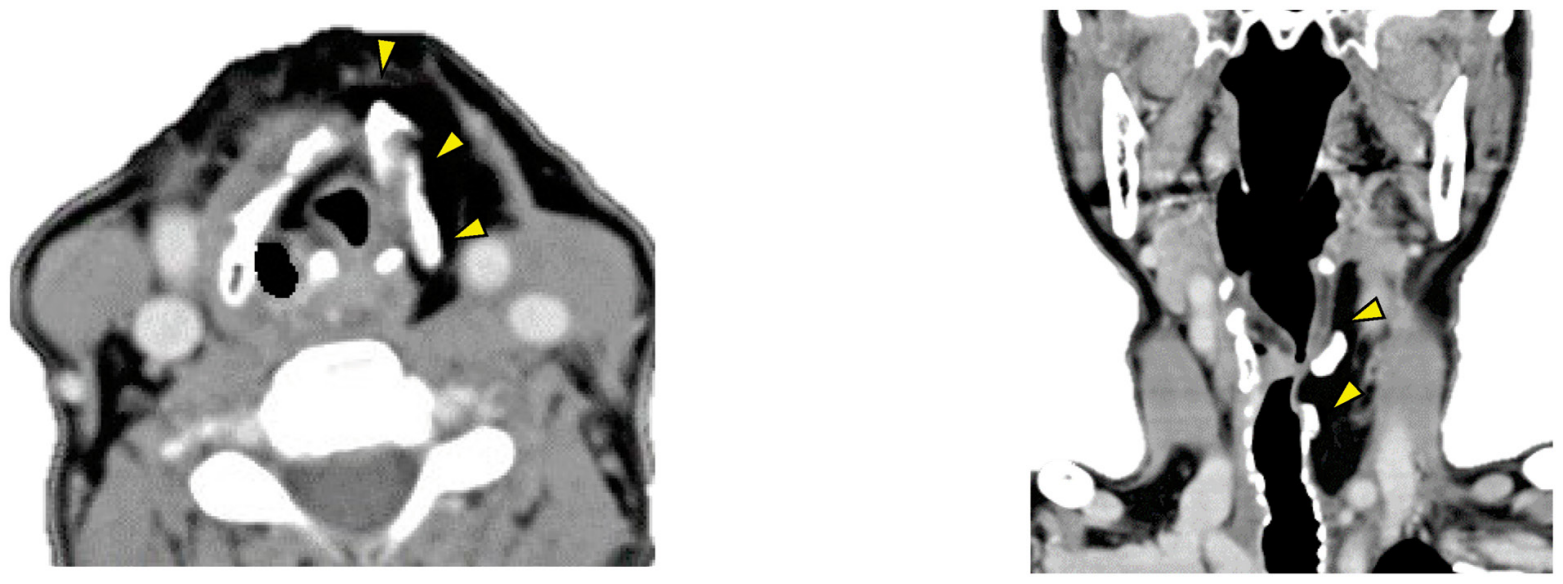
Post-operative computed tomography images show a patent laryngeal lumen. Arrowheads indicate rib cartilages.
